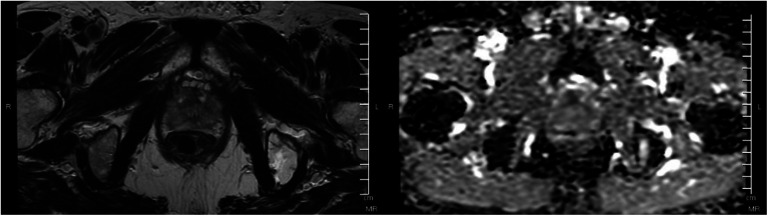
The MRI images of patient 2 (in Table 2). The T2W (T2-weighted) images and ADC (Apparent diffusion coefficient) images show the right apex anterior lesion. Targeted biopsy would have given this patient a category of clinically significant prostate cancer according to UCL1 criteria only. The SB resulted in a Gleason 4 + 3 biopsy which is the more influential factor leading to treatment. This patient had radical radiotherapy. SB, systematic biopsy; TB, targeted biopsy.
